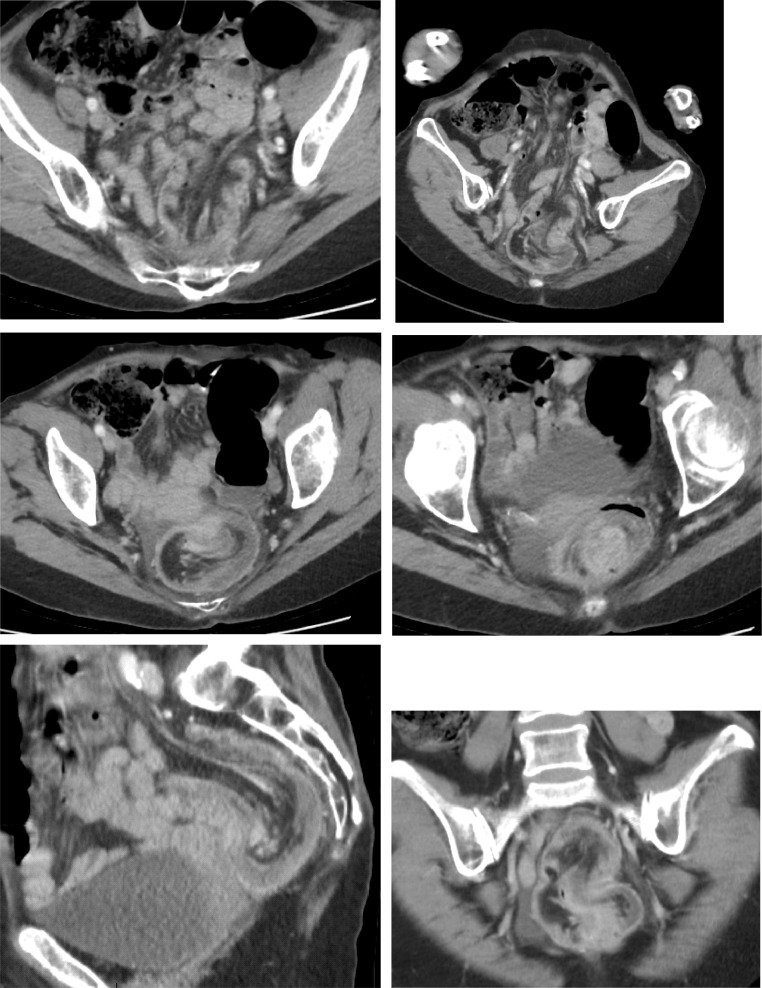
CT scan with axial, sagittal and coronal views respectively demonstrating intussusception of the sigmoid colon into the rectum.
